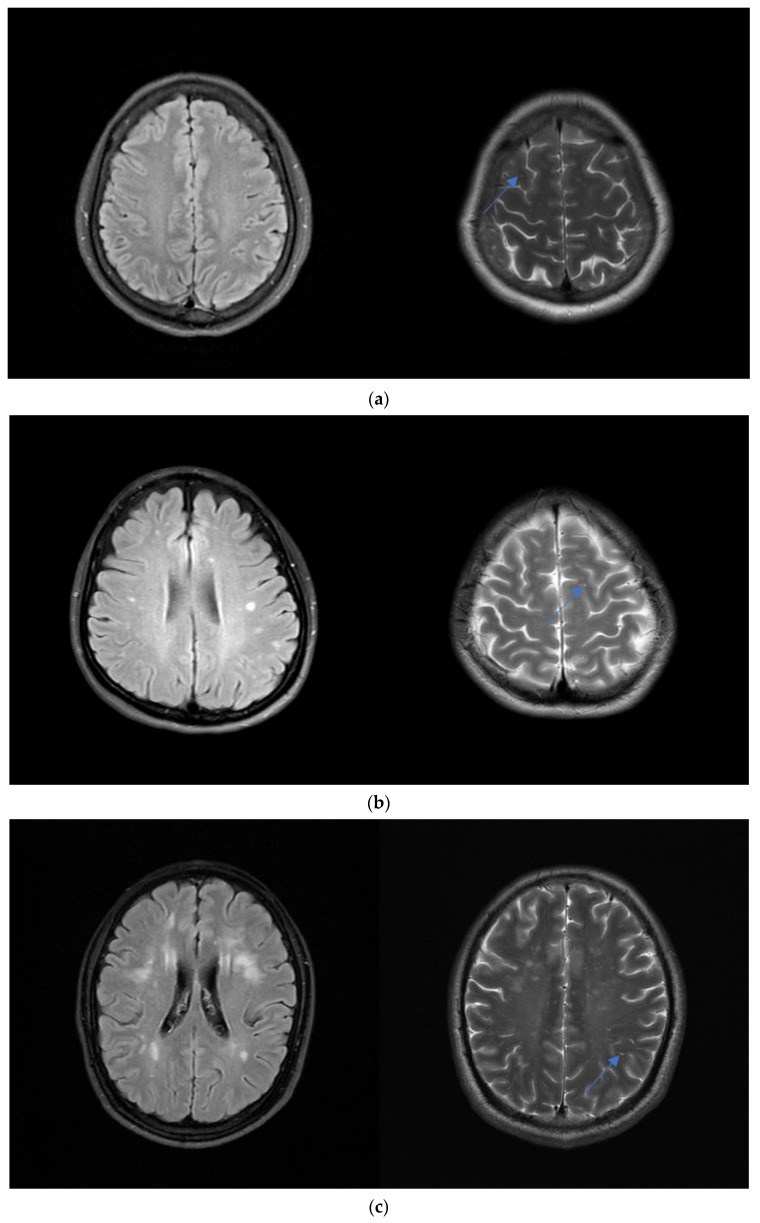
Levels of the severity of WMH. (a) A 23-year-old woman with a headache. Axial FLAIR MR images show mild DWMH. Axial T2-weighted images show grade 1 CSO-ePVS (blue arrow). (b) A 36-year-old woman with dizziness. Axial FLAIR MR images show moderate DWMH. Axial T2-weighted images show grade 2 CSO-ePVS (blue arrow). (c) A 45-year-old man with visual disturbance. Axial FLAIR MR images show severe DWMH. Axial T2-weighted images show grade 3 CSO-ePVS (blue arrow).
